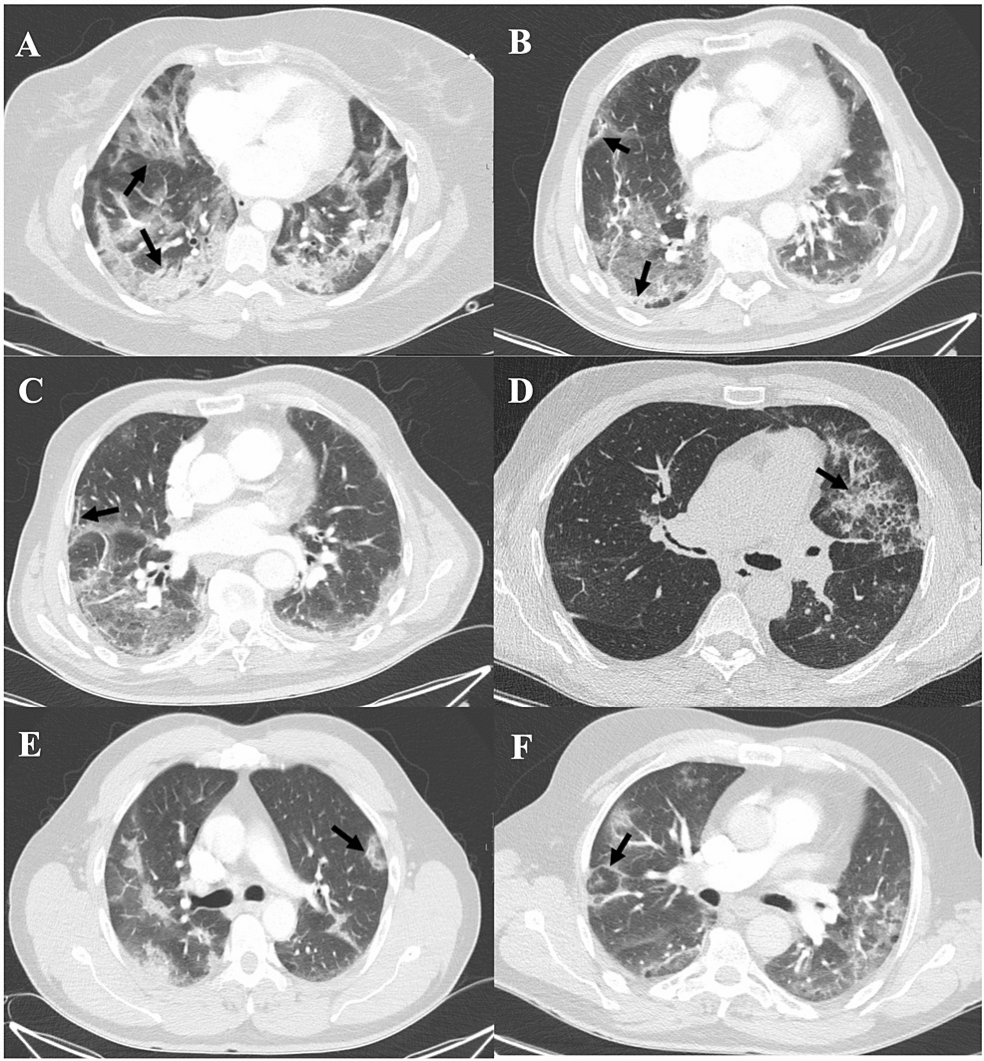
CT features of organising pneumonia in COVID-19 pneumonia patients (A) Bilateral subpleural and peribronchovascular consolidations (black arrow). (B) Subpleural linear consolidation (black arrow). (C) Parenchymal bands (black arrow). (D) Crazy paving pattern (ground-glass opacities with inter and intralobular septal thickening) (black arrow). (E) Perilobular opacities (ill-defined perilobular linear opacities, thicker than the thickened interlobular septa with an arch shape) (black arrow). (F) Reversed halo (attol) sign (central ground-glass opacity surrounded by denser consolidation of crescentic shape) (black arrow).
